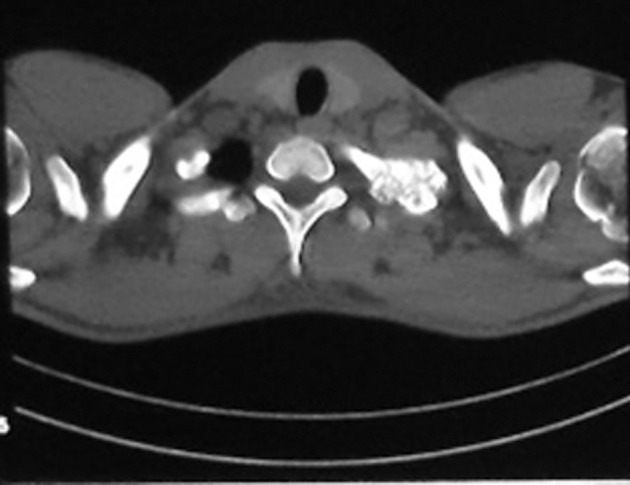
Non-contrast enhancement CT demonstrates an oval sclerotic, expansile lesion with spongy-form speckled calcification and a well-demarcated lesion involving the posterolateral side of the left first rib.
